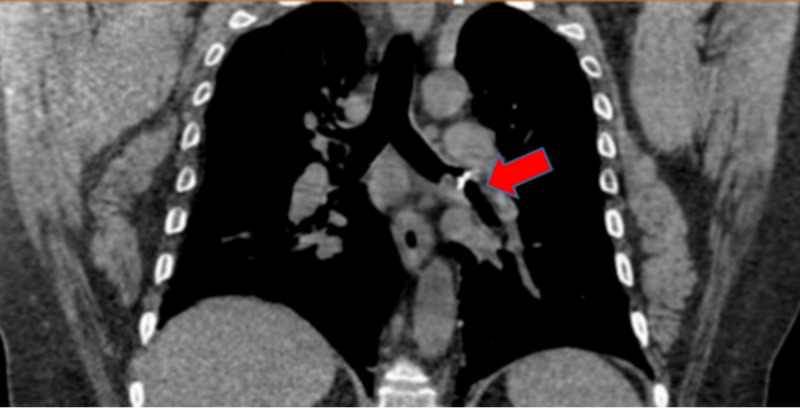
CT chest with 1 cm hyper-density (red arrow) at the bifurcation of the left main bronchus extending into the left upper lobe concerning for an aspirated FB, FB, foreign body
